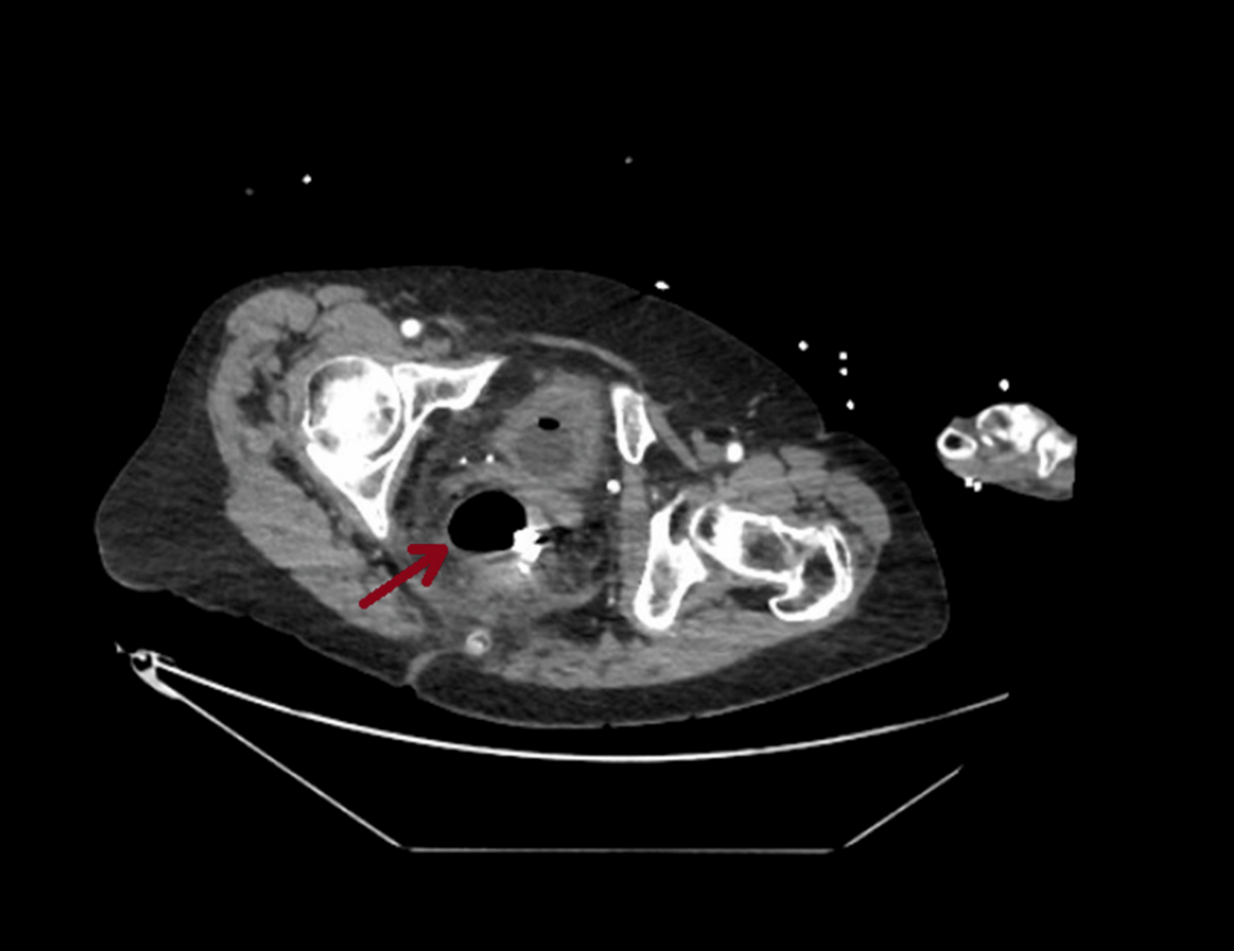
CT scan image showing edema and fat stranding around the rectum consistent with proctitis CT: computed tomography
